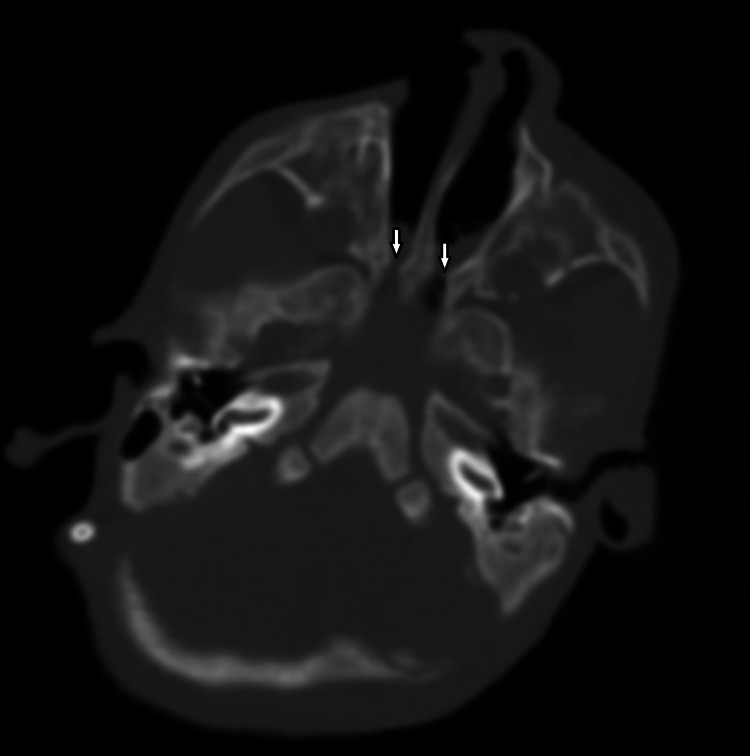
Axial computed tomography (bone window) of nose and paranasal sinuses showing bilateral choanal atresia (arrows).
